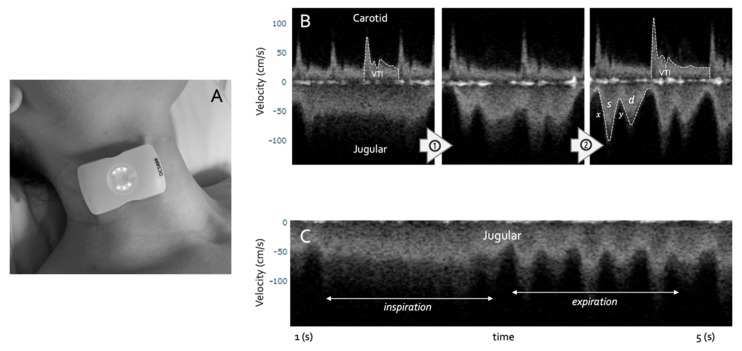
Simultaneous venous and arterial Doppler from a wearable ultrasound. (A) shows the wearable technology on a healthy volunteer. (B) shows the effect of rising preload from left to right (arrows 1 & 2 are ↑ preload). The jugular wave is amorphous indicating jugular collapse, as preload rises, the morphology of the jugular vein becomes more pulsatile and forms the s and d waves described above; the *x* and *y* refer to the pressure descents of the CVP. The carotid VTI also rises from left to right suggesting that the SV is rising with preload (e.g., quadrant 1 to 2, Figure 3). (C) shows just the jugular morphology from inspiration to expiration, intimating jugular collapse on inspiration.
